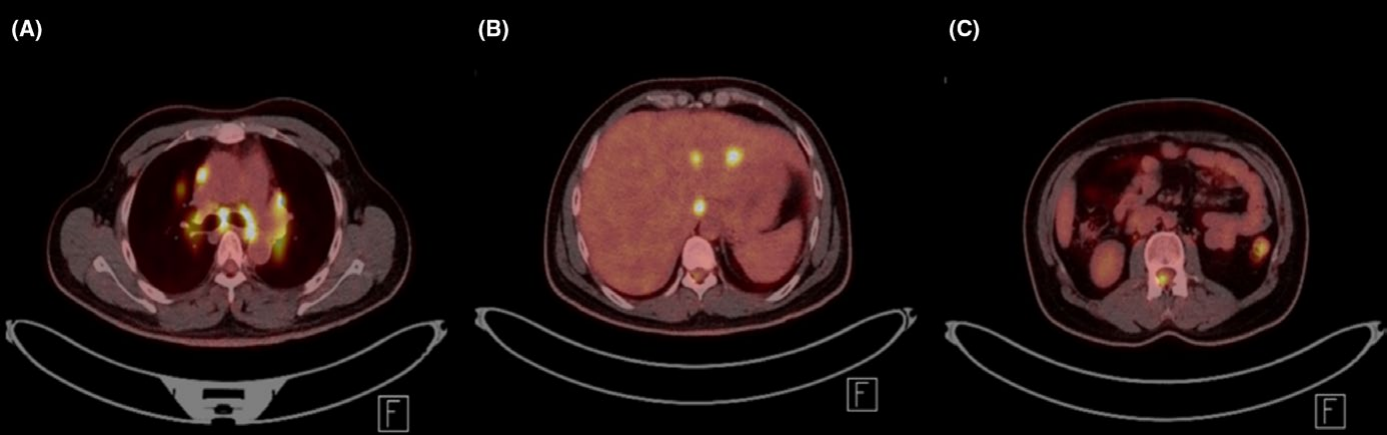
FDG‐PET Imaging. A, Intense metabolic activity associated with pathological mediastinal and bihilar lymphadenopathy. B, Multifocal FDG avid liver lesions. C, Intense focal tracer uptake within the spinal canal compatible with nodular enhancing MR lesions
